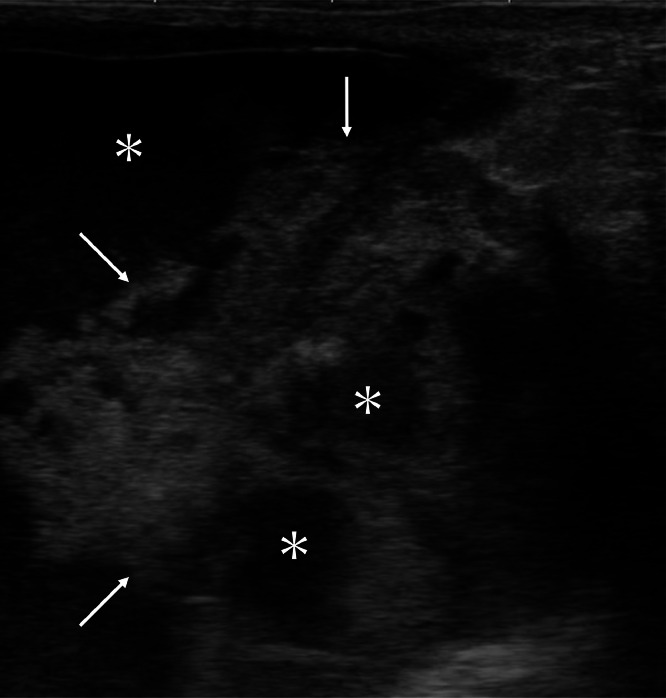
Ultrasound findings. Ultrasound showed hyper echoic mass components (arrows) and cystic parts (asterisks).
